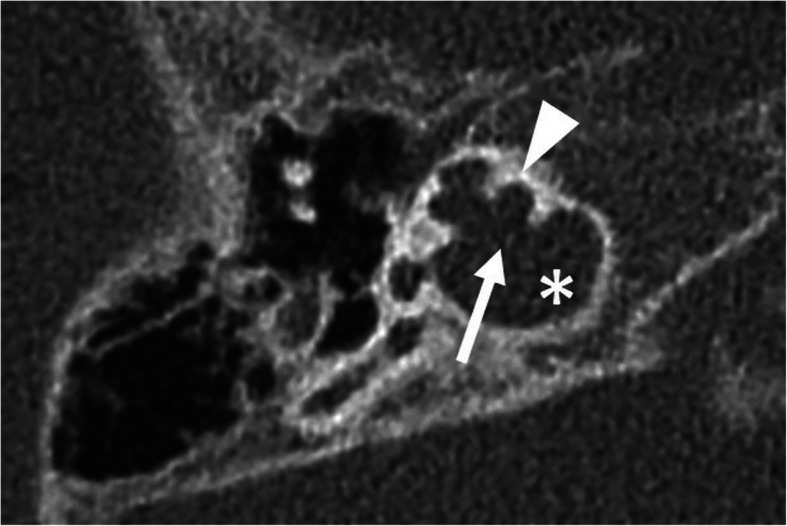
A 1-year-old male patient, with bilateral severe SNLH from birth. HRCT axial image shows incomplete partition type III with empty cochlea with preserved interscalar septa (arrowhead). Modiolus and bony separation of the cochlea and internal auditory canal are absent (arrow). The cochlea is placed directly at the lateral end of the internal auditory canal (asterisk)
